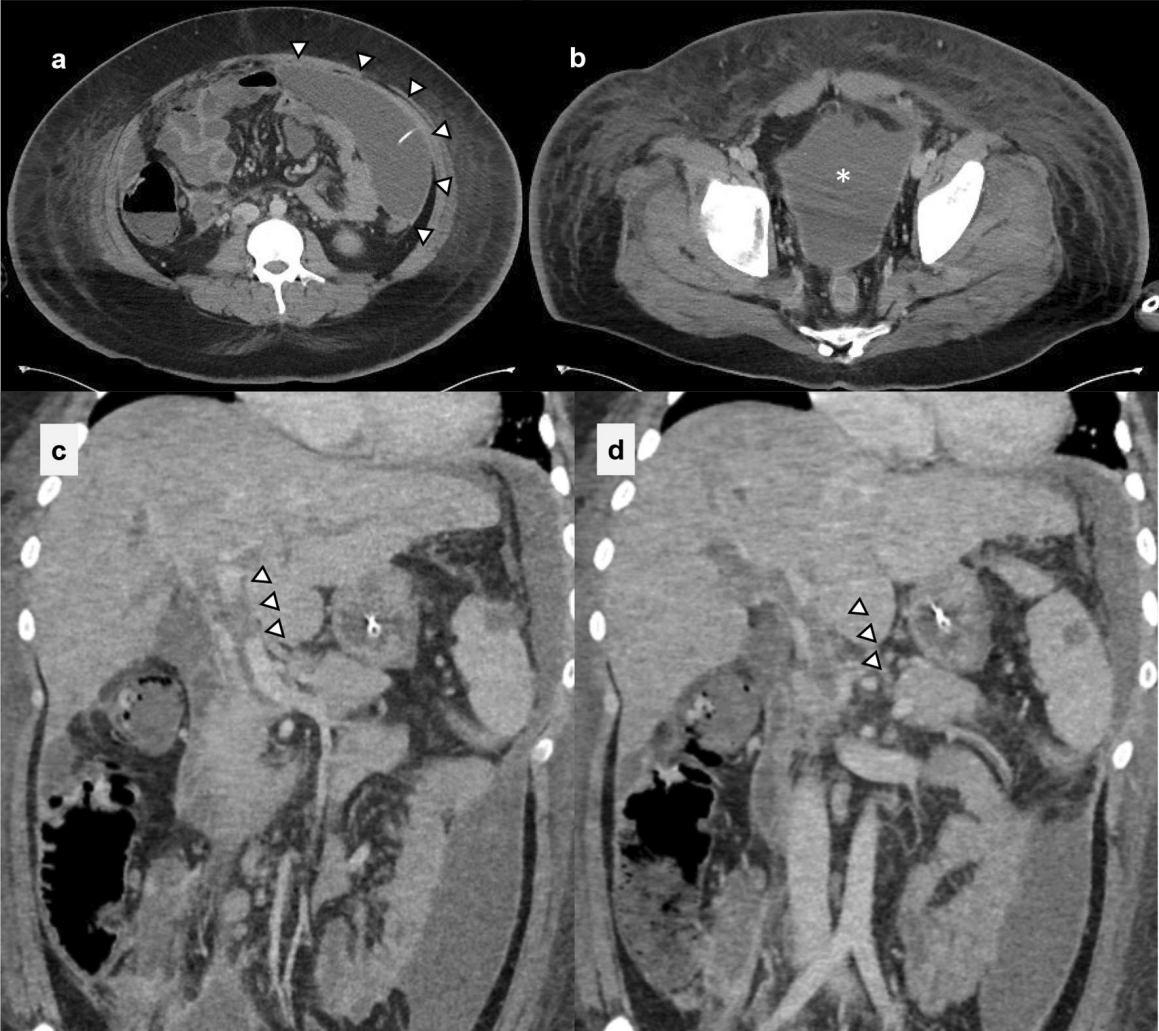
CT on day 10, when CRP levels started rising again, demonstrated a an abdominal paracentesis into an intra-abdominal abscess (arrowheads). b The abscess extended to Douglas's pouch (asterisk). c and d a low-density area in the portal vein of the sagittal sections (arrowheads). CT: computed tomography; CRP: C-reactive protein
